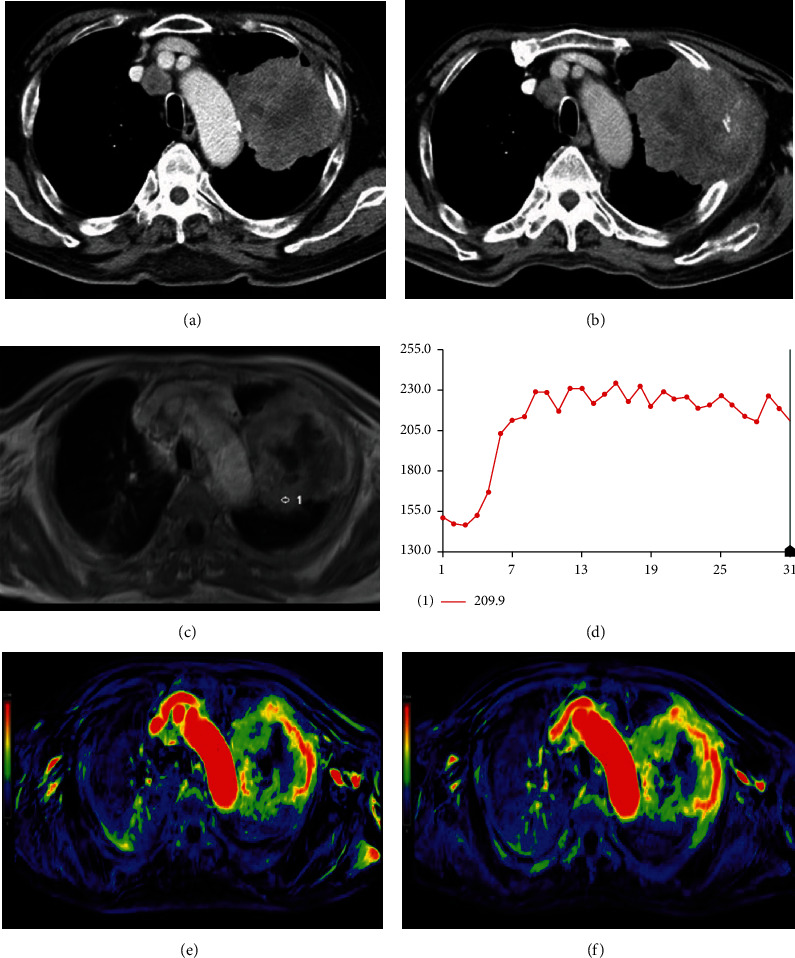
Axial CT scan of a 68-year-old male with lung squamous cell carcinomas at the left upper lobe before (a) and after (b) three months of chemotherapy. At the maximum transverse dimension, about 12.0% increase of the tumor size was observed, and the patient was categorized as a nonresponder. The DCE-MRI map (c) and TIC map (d) are shown. Images of color MR PEI mapping before (e) and after (f) one week of the first course of chemotherapy showed not significant increase in tumor perfusion (the value was 914 vs. 1078).
